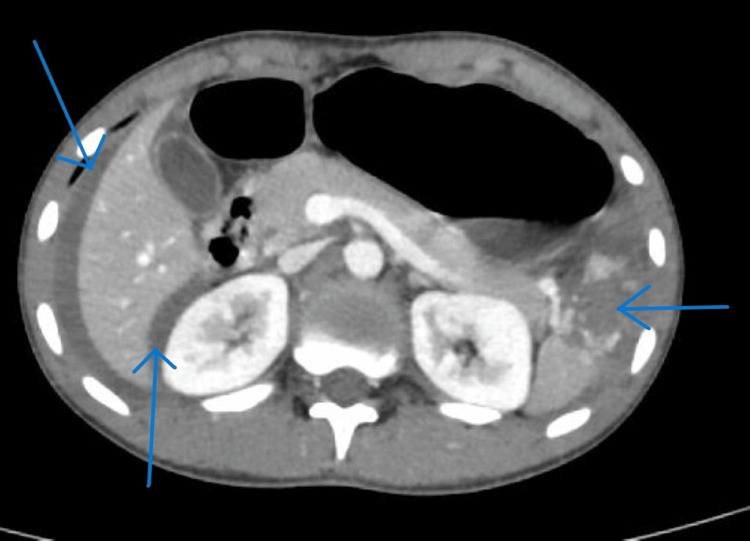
Contrast-enhanced CT of the abdomen showing splenic laceration. Contrast-enhanced CT abdomen (axial view, portal venous phase; slice thickness 5 mm; window/level 350/50 HU) showing low-attenuation perisplenic fluid collections and dependent hemoperitoneum (blue arrows) consistent with splenic laceration (AAST grade IV). The liver (L), spleen (S), and kidneys (K) are shown for anatomical orientation. AAST: American Association for the Surgery of Trauma; HU: Hounsfield unit
